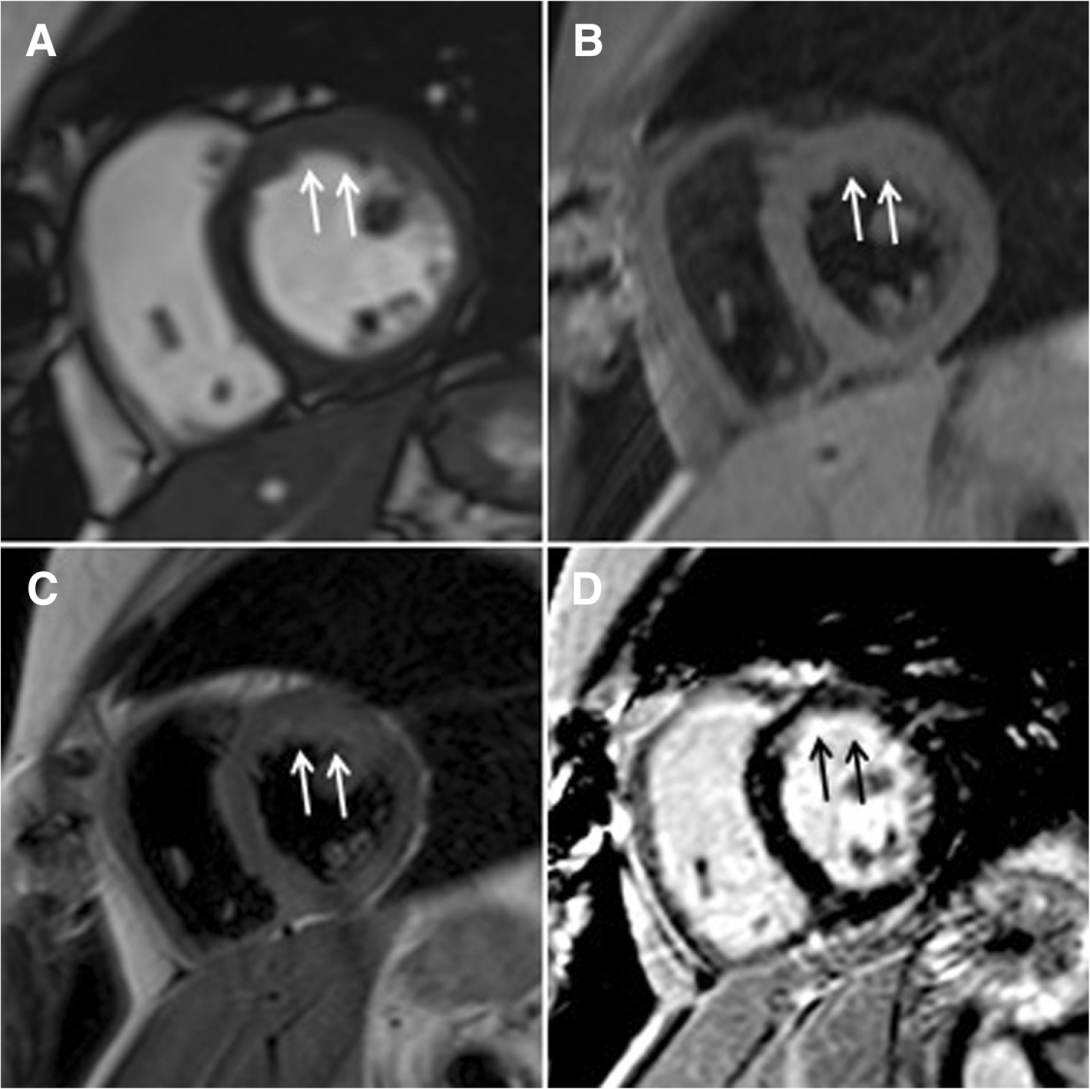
The CMR study after 7 cycles of chemotherapy treatment. Complete response in cine (a), T1-weighted (b), T2-weighted (c) and LGE (d) images. LGE = late gadolinium enhancement
